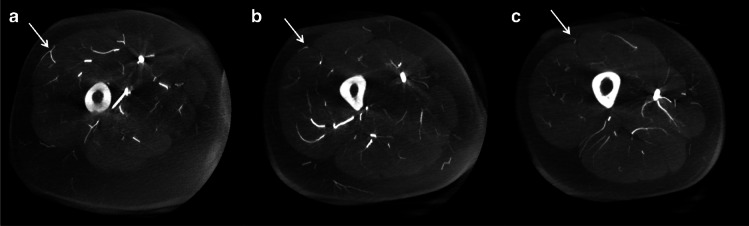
Flat-Panel Computed Tomography (FPCT) Imaging of a thigh for ALT flap perforator mapping. White arrow points at the point of fascial emergence (a–c).
